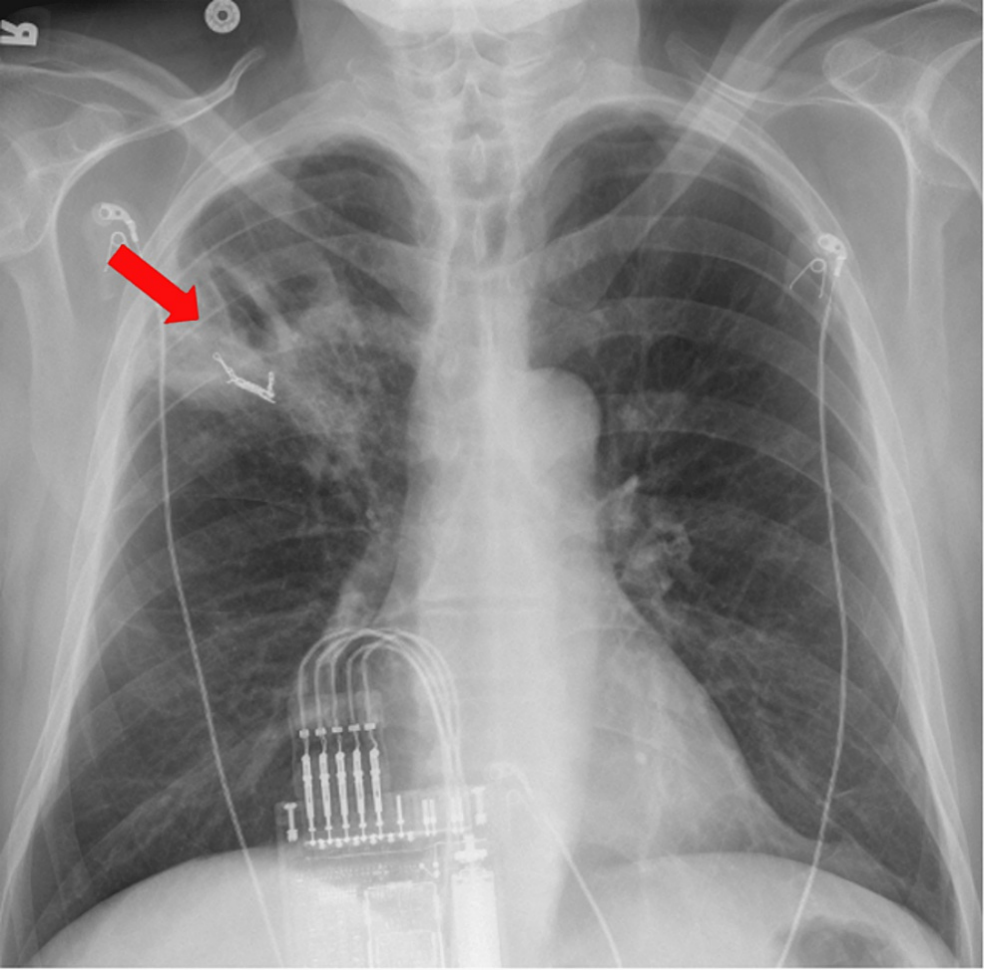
Repeat chest radiograph on hospital admission day 6 shows interval decrease of the right upper lobe cavitary lesion as well as coils from the embolization (red arrow).
